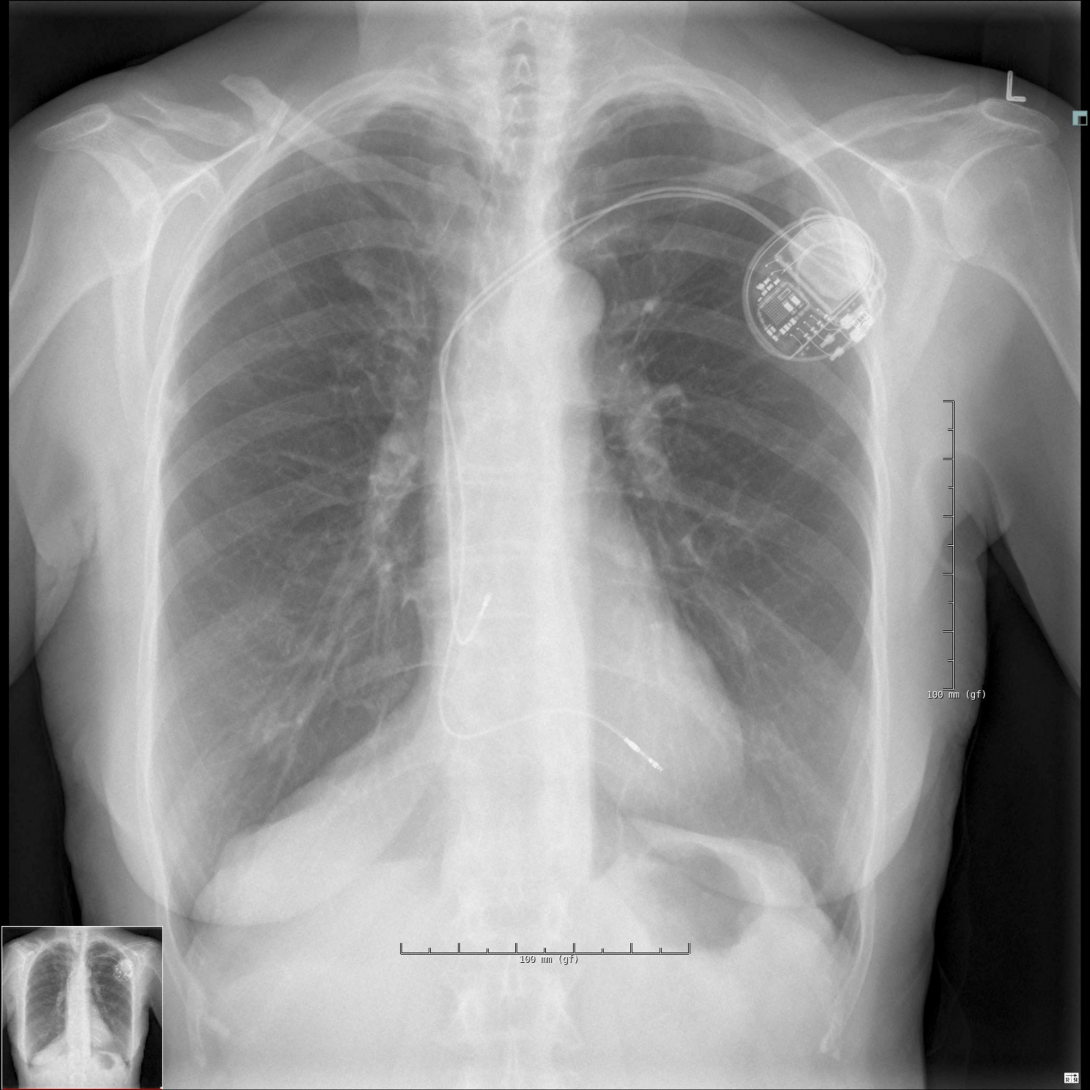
Chest X-ray has normalized 13 days after implantation of the pacemaker.
